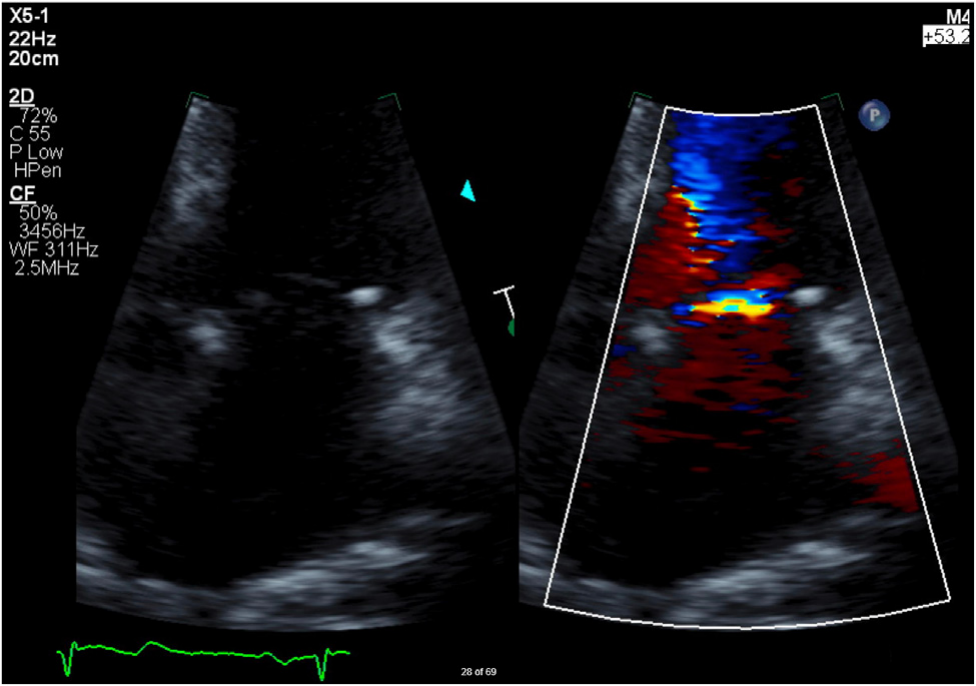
Nonenhanced 2D TTE, zoomed-in apical four-chamber view, without (*left*) and with (*right*) color flow Doppler in systolic phase, demonstrates a distinct horizontal line of color signal across the mitral annular surface of the left atrium, without evidence of any further regurgitant jet.
